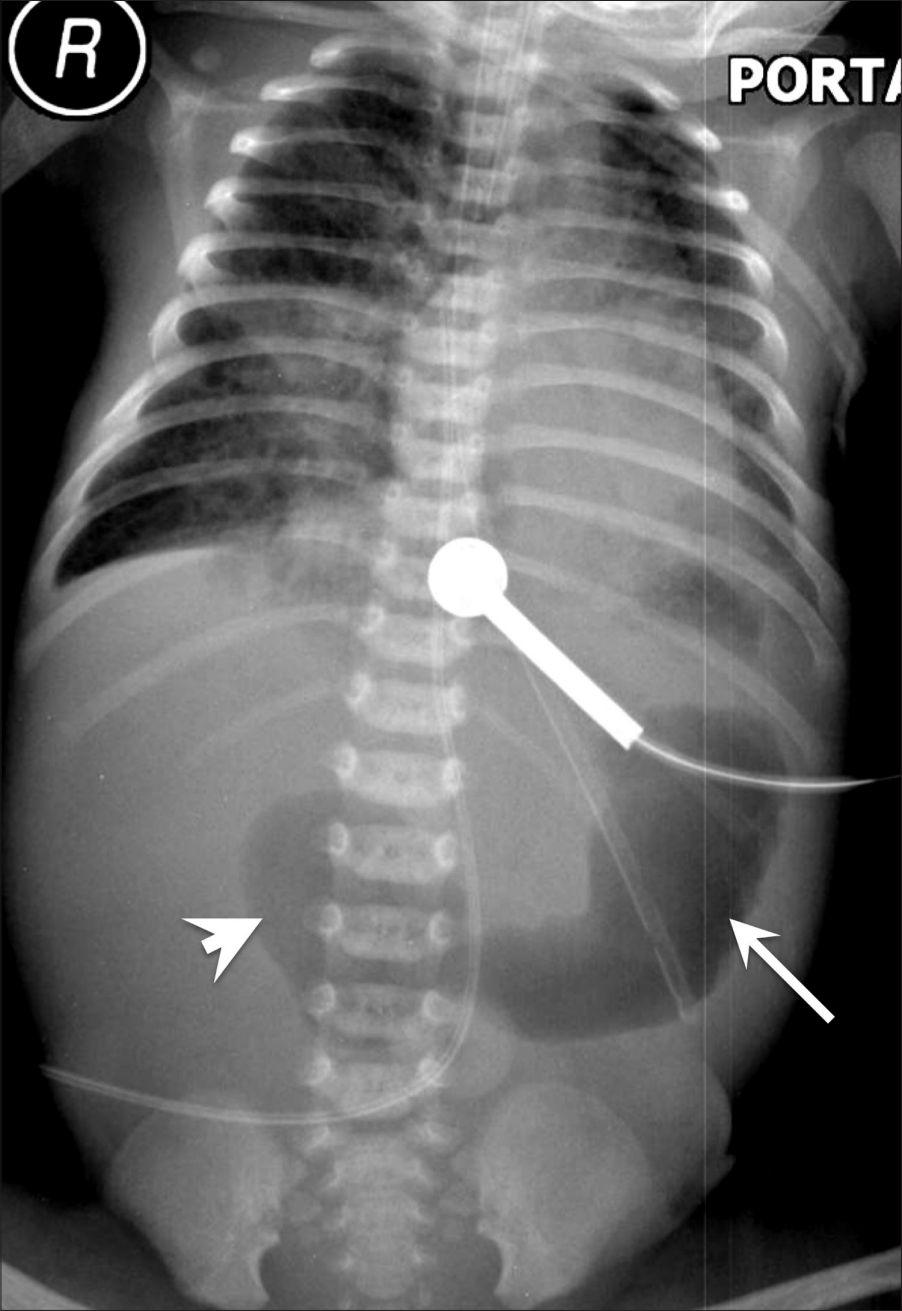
Duodenal atresia: Frontal radiograph of the chest and abdomen on day 2 shows a dilated stomach and proximal duodenum, with the classical ‘double bubble’ sign. Note the absence of gas shadows in the rest of the abdomen and the bilateral patchy basal pneumonia due to aspiration
